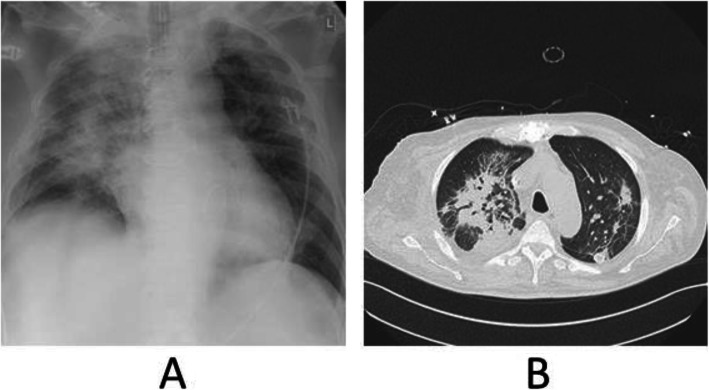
Suspicion of COVID-19 Infection. a Thoracic Radiography; b Thoracic CT-scan
